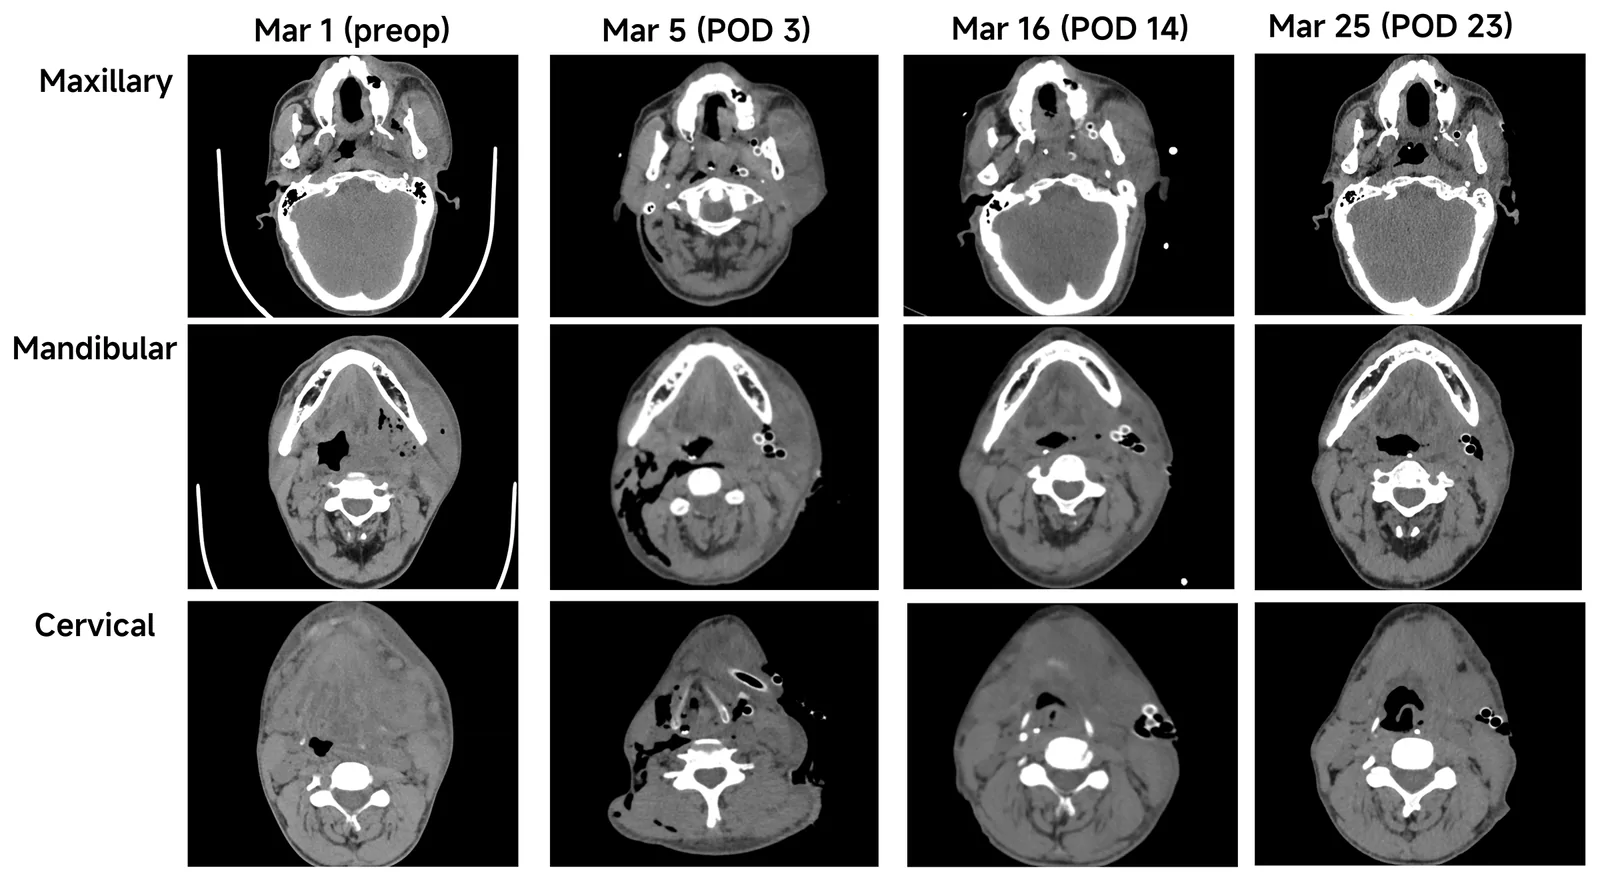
Serial cervical CT at the maxillary (row 1), mandibular (row 2), and cervicothoracic (row 3) levels. Column A (March 1, preop): diffuse left maxillofacial soft-tissue swelling, obliteration of fat planes, and gas-forming multi-space abscesses. Column B (March 5, postoperative day 3): extensive subcutaneous emphysema of the bilateral cervicofacial soft tissues extending to the thoracic inlet. Column C (March 16, postoperative day 14): marked resorption of gas and regression of the space infections. Column D (March 25, postoperative day 23): near-complete resolution with minimal residual gas. preop: preoperative; POD: postoperative day.
